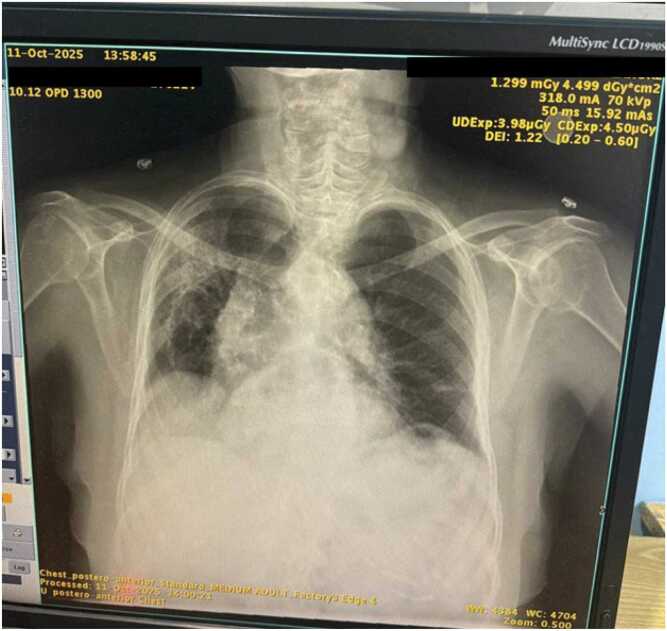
Chest X-ray on admission (11 October 2025): shows bilateral patchy consolidations predominantly in lower zones, consistent with severe pneumonia.
